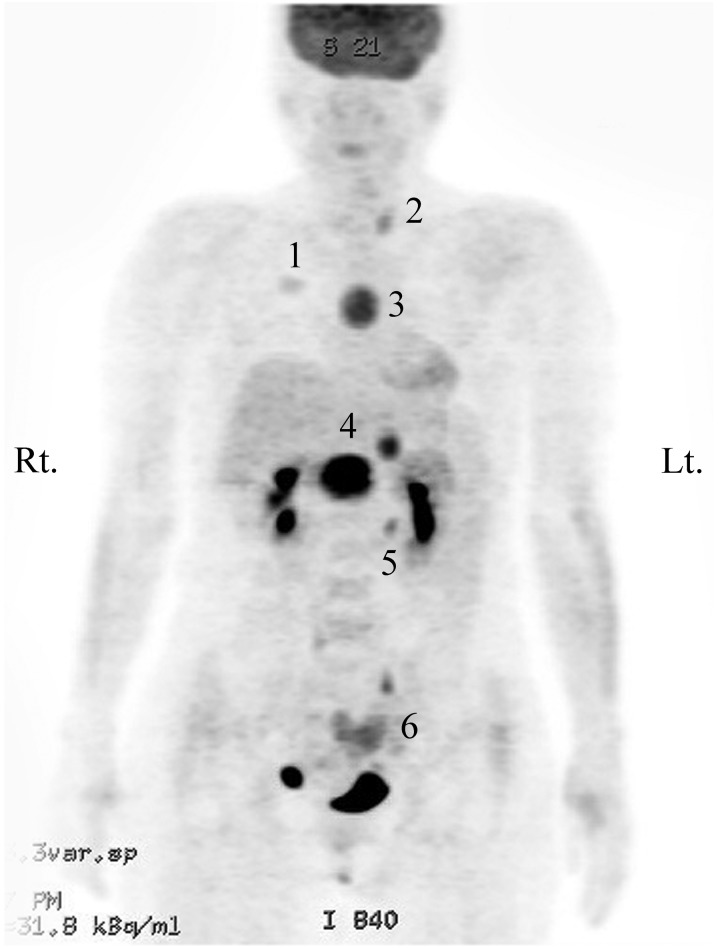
Findings of positron emission tomography-computed tomography (PET-CT). PET-CT showed (1) a metabolically mildly active right breast lesion compatible with the proven right breast cancer, which was proven by an ultrasound-guided core needle biopsy (2) a metabolically mildly active left thyroid lesion, which was suspicious for thyroid cancer, (3) a metabolically active prevascular (ascending paraaortic) nodal lesion, (4) metabolically active upper abdominal nodal lesions (left gastric lymph node and adjacent to hepatic and splenic artery), which were suspicious for metastatic nodal lesions or a primary pancreas tail mass (4), (5) suspicious left adrenal metastasis and (6) mild endometrial uptake, which was suspicious for myoma or endometrial malignancy.
